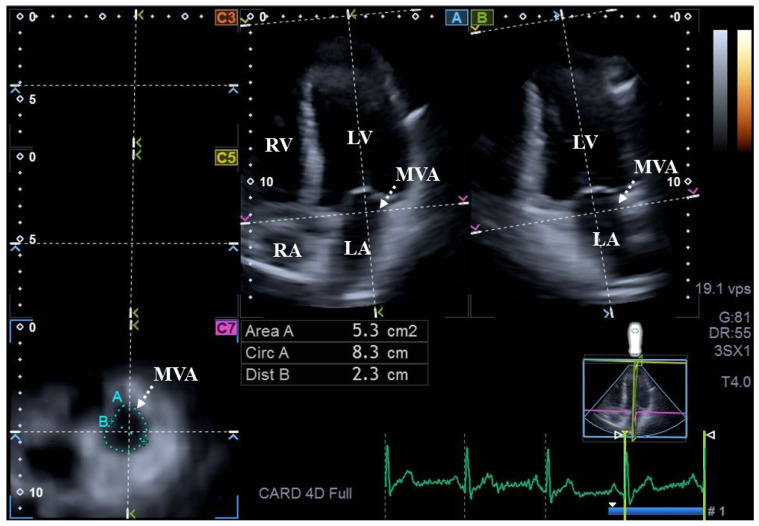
Three-dimensional speckle-tracking echocardiography-derived assessment of mitral annular dimensions in a healthy adult in end-diastole: (A) apical four-chamber and (B) apical two-chamber long-axis views and cross-sectional view (C7) of the mitral annulus optimized on A and B images. Mitral annular plane is indicated by white dotted arrows. Abbreviations. Area = mitral valve annular area, Circ = mitral valve annular perimeter, Dist = mitral valve annular diameter, LA = left atrium, LV = left ventricle, RA = right atrium, and RV = right ventricle.
